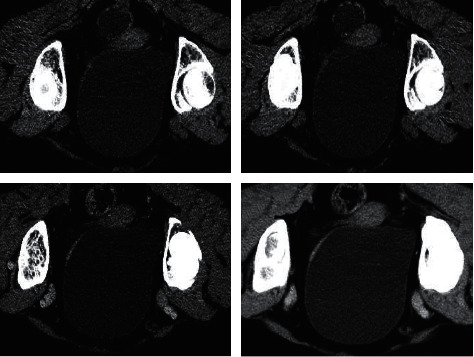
The CT image of a 63-year-old male patient. The general condition was good, and he was admitted after 8 days of hematuria.
